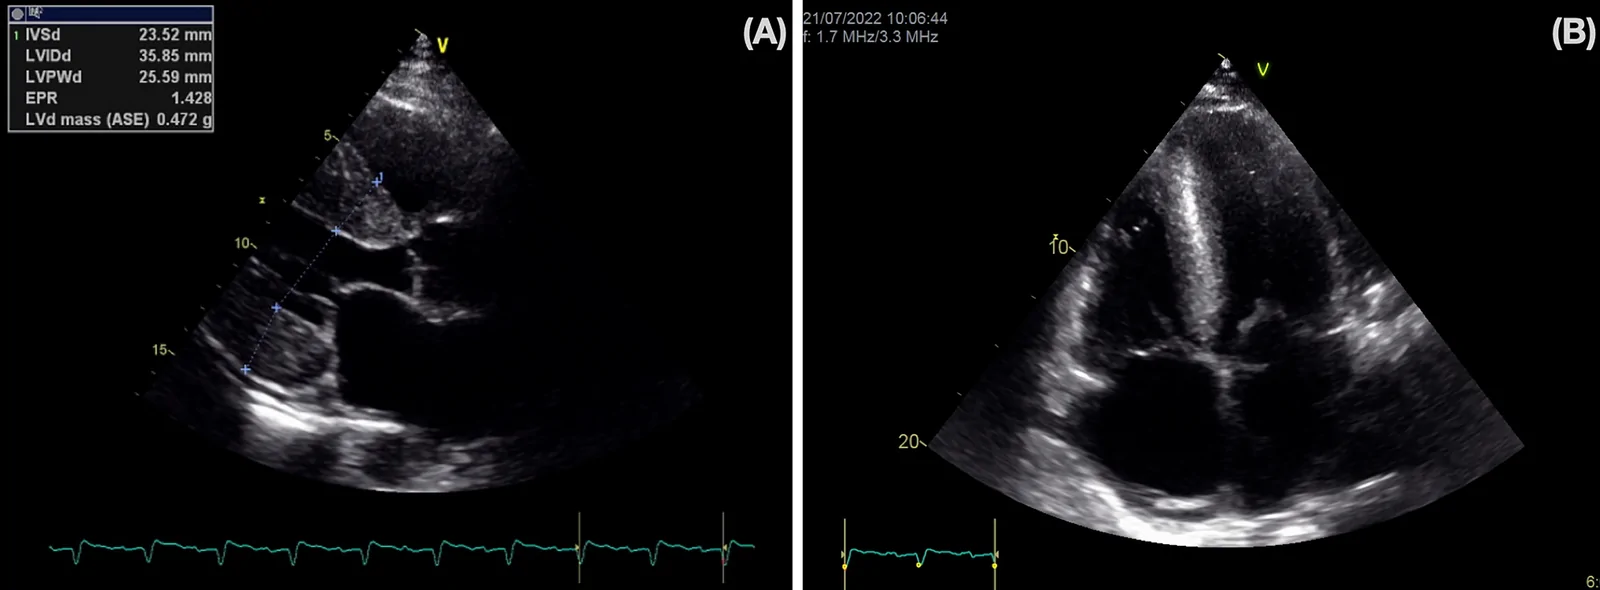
Transthoracic echocardiogram parasternal long axis (A) and apical four chamber view (B), revealing moderate concentric left ventricular hypertrophy with preserved biventricular systolic function
